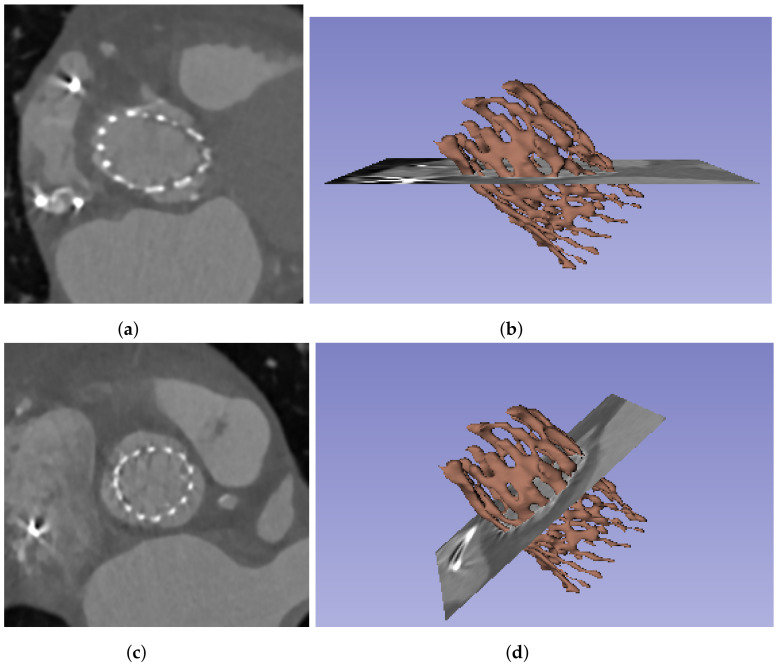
Original and orthogonal views of the CT images captured from a patient with an Allegra of 27 mm implanted. (a) Axial view of the original image slices, (b) prosthesis 3D reconstruction, with the axial view of the original images, (c) orthogonal view of the prosthesis, after the image transformation, (d) prosthesis 3D reconstruction, with a plane orthogonal to the prosthesis principal axis.
